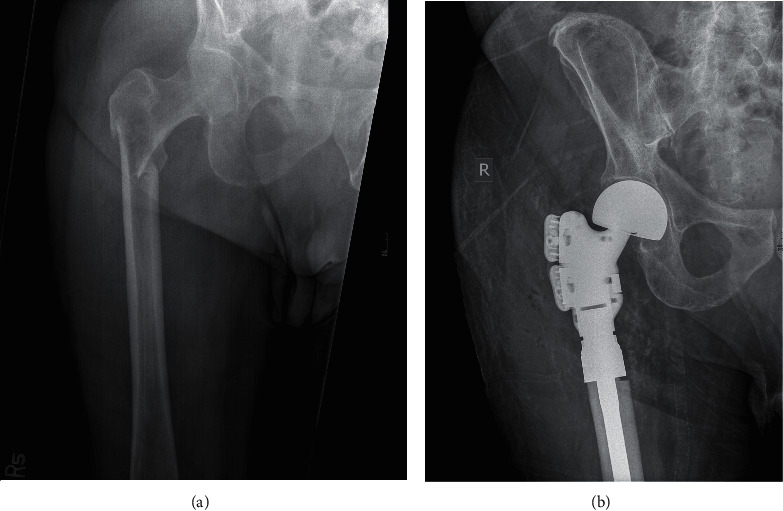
Injury and postoperative radiographs of the pathologic subtrochanteric femur fracture in the patient described in Figure 2. Twelve days after the routine surveillance CT, the patient sustained a pathologic subtrochanteric femur fracture after a fall from a standing height (a). He subsequently underwent proximal femoral replacement by an oncology fellowship-trained surgeon (b).
